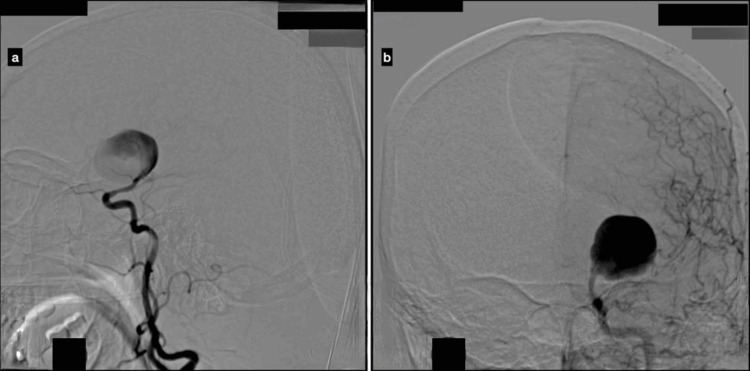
Lateral and Towne’s views of a patient who presented with symptoms of ICP and mass effect on NCCT of the brain. Left ICA injection showing a giant MCA aneurysm filling without opacification of the distal MCA branches. a: lateral view of the left ICA run; b: Towne's view of the left ICA run. ICA: internal carotid artery; MCA: middle cerebral artery; ICP: intracranial pressure; NCCT: non-contrast CT
